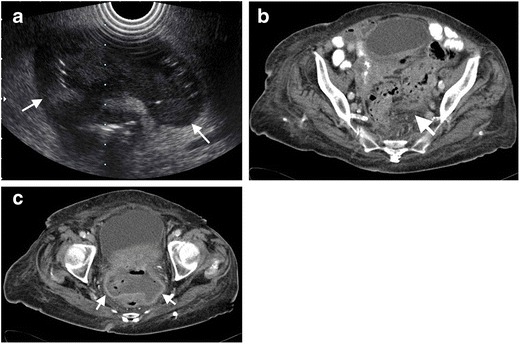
Abscess resulting from a diverticulitis in an 85-year-old female. a Endovaginal US demonstrates a fluid collection with gas content and wall thickening (*arrows*) representing abscess. b Axial contrast-enhanced CT image reveals diverticulitis with edematous sigmoid colon, multiple diverticulum and surrounding fat stranding (*arrow*). c Axial contrast-enhanced CT of the same patient demonstrates a pelvic abscess (*arrows*) with enhancing wall and air content in a Douglas pouch
